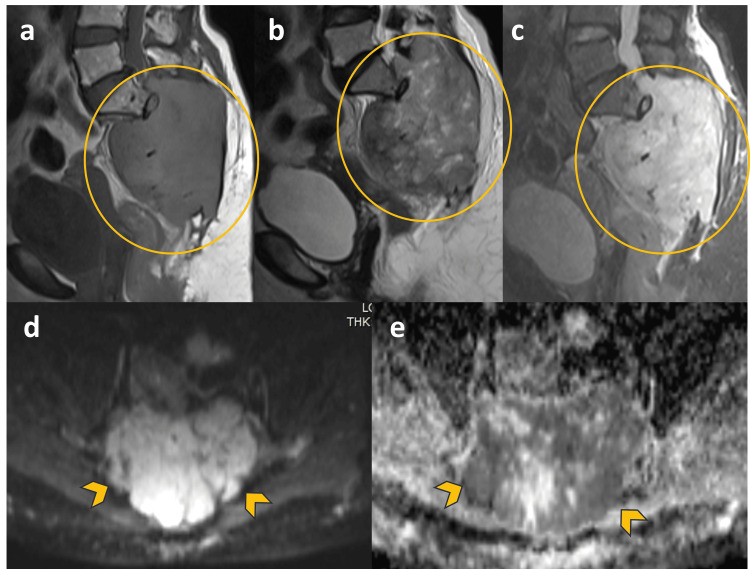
MRI signal characteristics of sacral chordoma MRI demonstrates a lobulated sacral mass with iso- to hypointense signal on T1-weighted imaging (a) and heterogeneous hyperintensity on T2-weighted and STIR sequences (b, c). The lesion fills the sacral canal and extends into the presacral space (circled area). Diffusion-weighted imaging (d) demonstrates moderate restricted diffusion with corresponding low signal on ADC mapping (e) (arrowheads).
